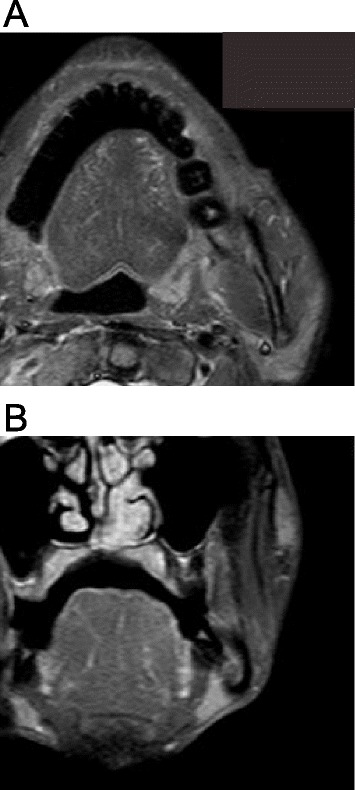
MRI assessment after treatment. STIR (a) and contrast-enhanced T1-weighted imaging (b) at 22 months after treatment show no apparent abnormal lesion in the corresponding area
